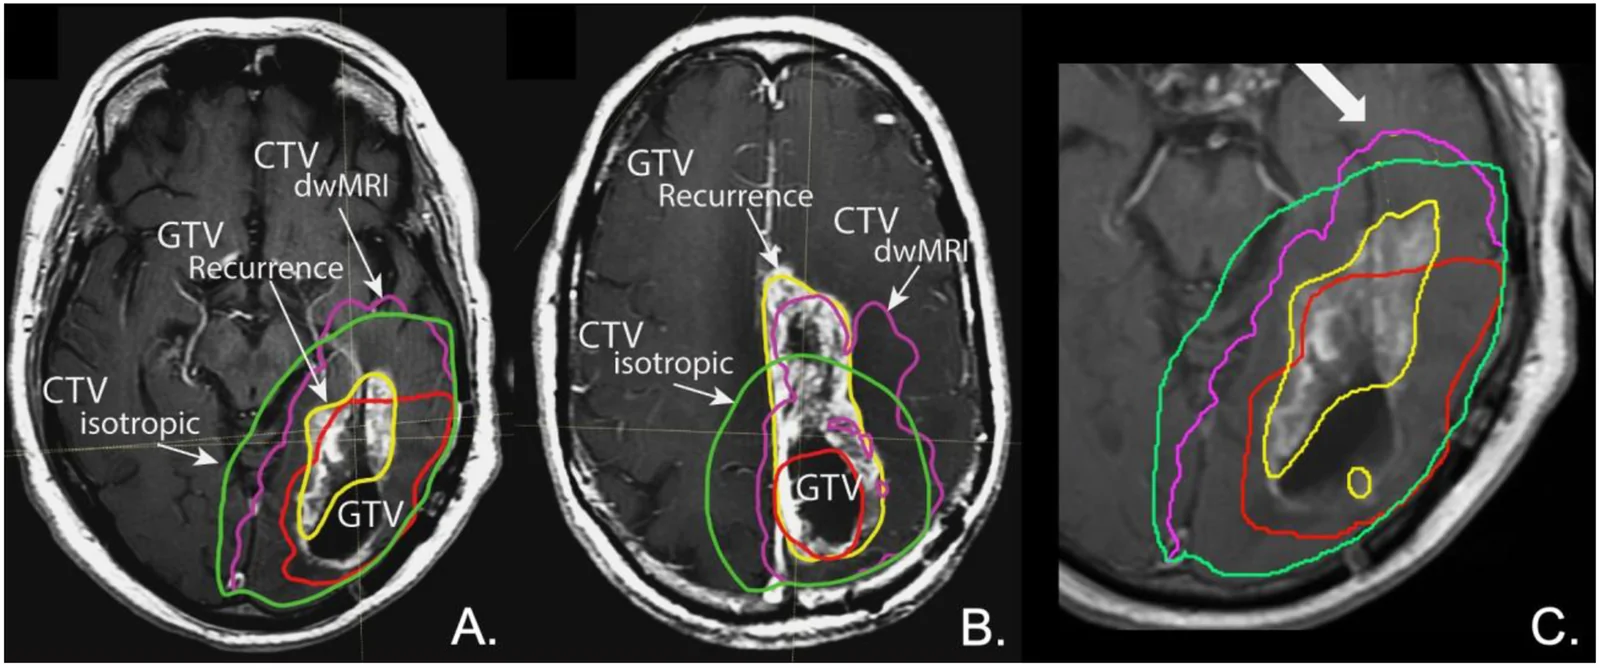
Comparison of the isotropic CTV_isotropic_ (in green) and 2-cm margin anisotropic CTV_tract_ (in magenta). The GTV to CTV expansions in relation to the radiographic recurrence are displayed for 3 different patients of the studied cohort. (A) Example of an infield recurrence (patient 3). (B) Example of a marginal recurrence (patient 7). (C) T1 post-contrast at time of recurrence for patient 12 from Table 1, demonstrating recurrence volume (yellow) covered by both 2 cm CTV_tract_ (magenta) and CTV_isotropic_ (green), but with 2 cm CTV_tract_ overall sparing more brain. The original GTV volume is in red. Arrow indicates recurrent tumor extension along inferior longitudinal fasciculus where the CTV_tract_ captures the overall direction of the progression
